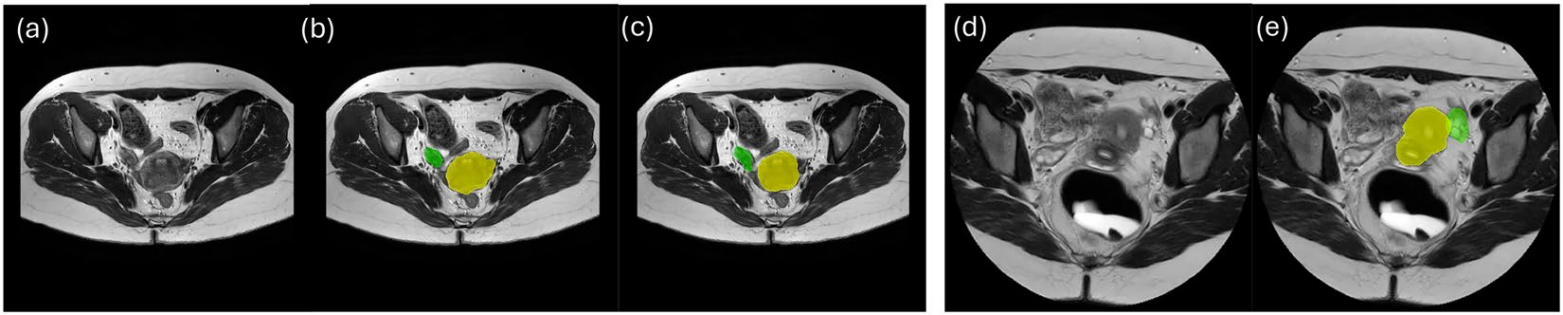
Examples of MRI scans for two Datasets. (a) T2-weighted MRI and (b,c) the corresponding uterus (yellow) and ovaries (green) labels from different raters for the first dataset. (d) T2-weighted MRI and (e) the corresponding uterus and ovaries labels for the second dataset.
